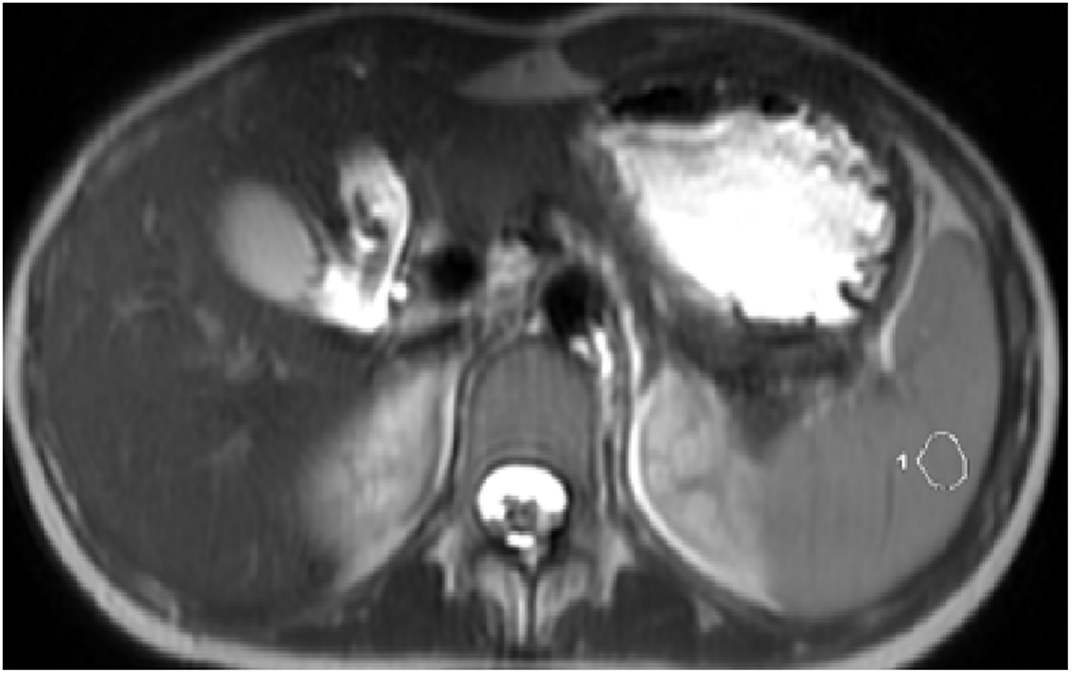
Demonstration of spleen intensity calculation on the axial T2-weighted image.
